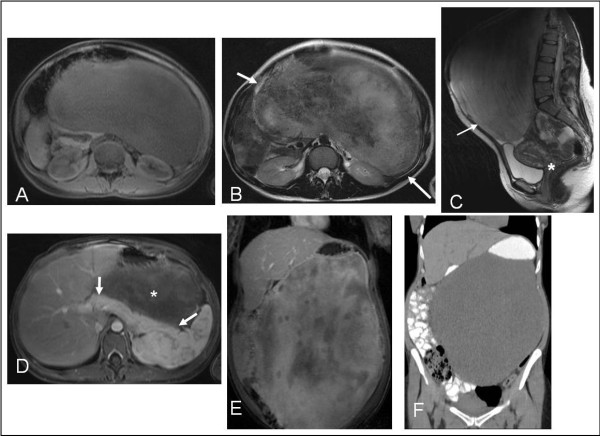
Radiological images. (A) Unenhanced T1-weighted MRI shows large, almost homogeneous, well-defined, intermediately low signal intensity mass, occupying the abdominal cavity, displacing solid organs and bowel loops. (B, C) T2-weighted MRI depicts heterogeneously hyperintense mass (arrows), with prominent areas of low signal intensity and unrelated to the bladder and uterus (asterisk). (D, E) Gadolinium-enhanced T1-weighted MRI shows no intervening fat planes between homogeneously enhancing pancreas (arrows) and slowly and heterogeneously enhancing tumour (asterisk), with intervening low signal intensity areas. (F) Unenhanced CT demonstrates the mass displacing opacified bowel loops.
